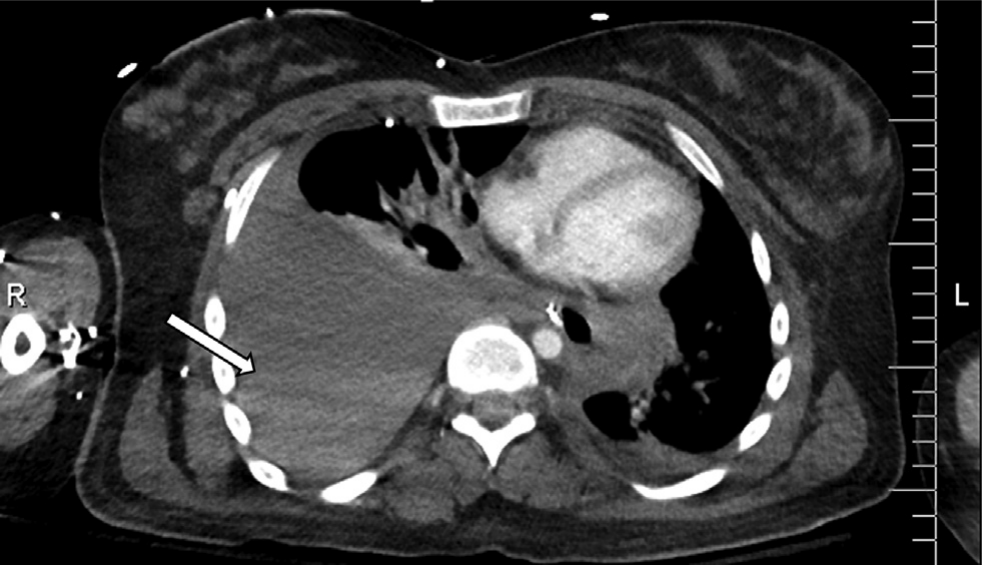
CT of chest showing large right pleural effusion with layering hyperdensity compatible with hemothorax.
